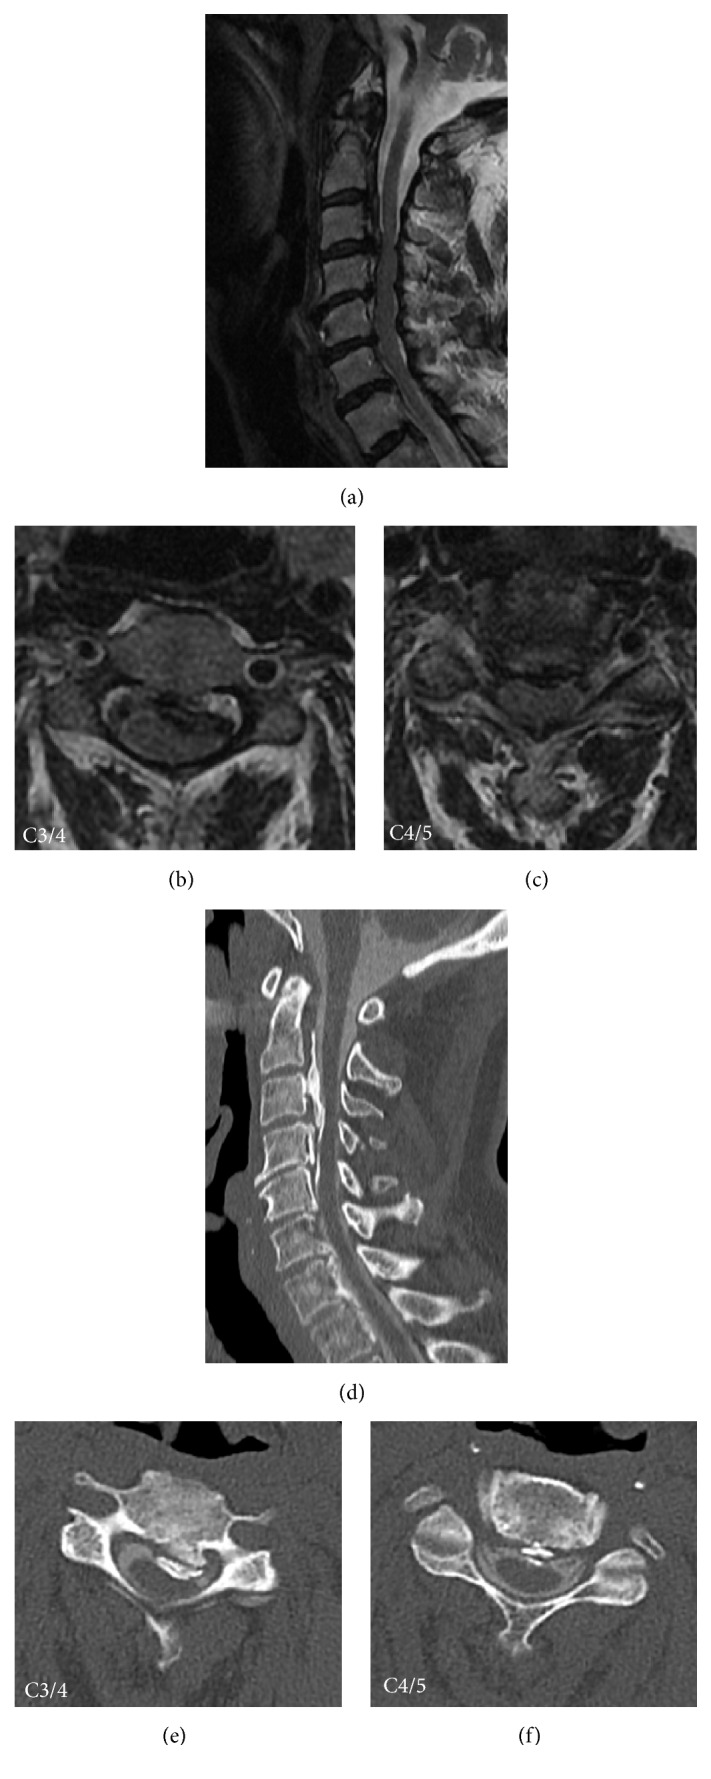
Preoperative images. Preoperative MRI (a, b, and c) and CT myelogram (d, e, and f) showed mixed-type OPLL compressing spinal cord, especially at C3-4 (OPLL canal occupying ratio: 50%). MRI showed no obvious cord signal change and cord enlargement (a, b, and c). The anterior-posterior diameters of right and left C4/5 foramen were 3.8 mm and 3.5 mm, respectively (f).
